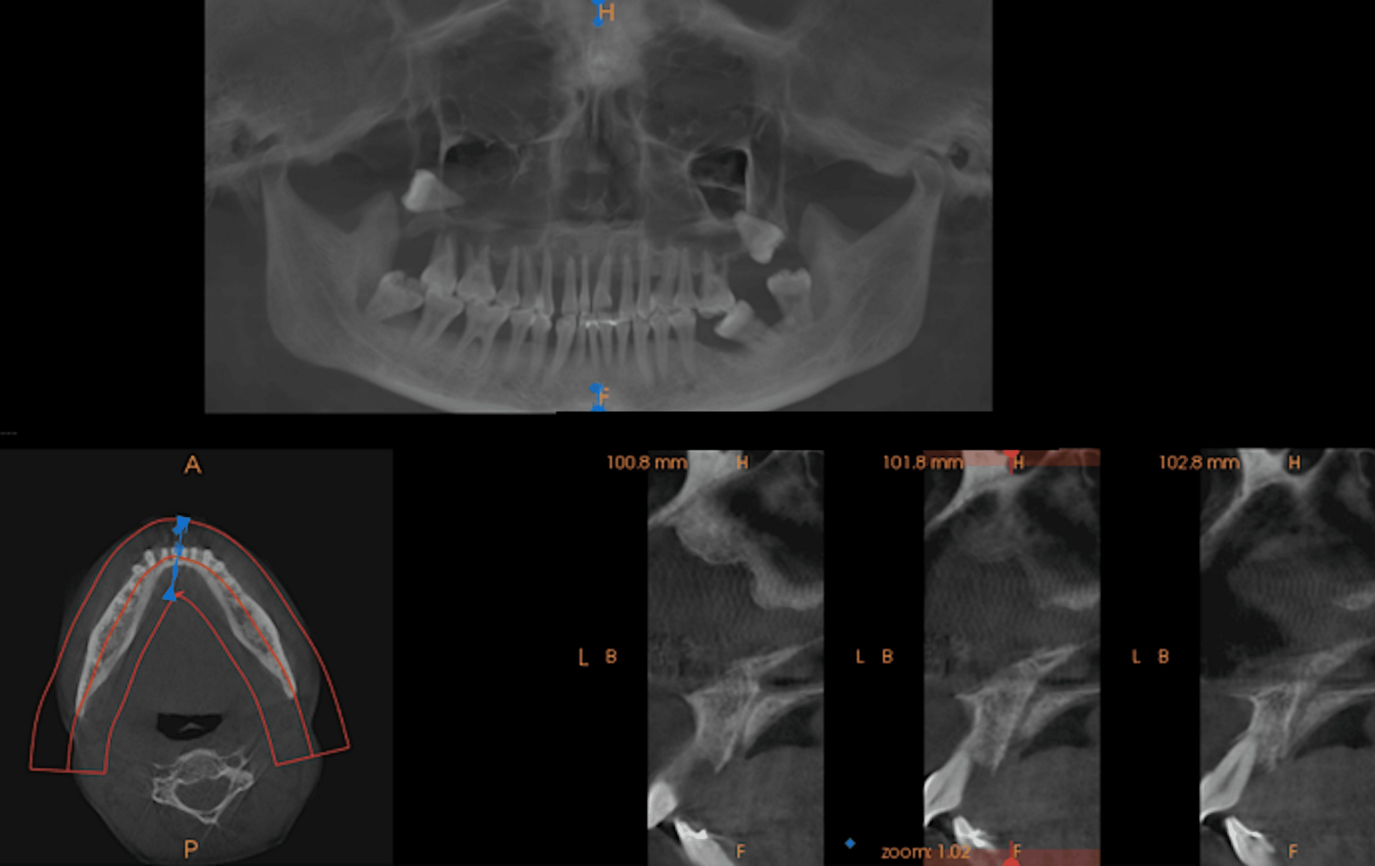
The CBCT revealed generalized bone resorption and root resorption in teeth 16 and 17. The floor of the maxillary sinus was not traceable, with impacted teeth 18 and 28, with root portions extending into the maxillary sinus. A generalized interdental bone loss was observed in the mandible, with severe bone loss in teeth 37, 38, 47, and 48, and displaced teeth 37, 38, and 48 CBCT = Cone-Beam Computed Tomography
